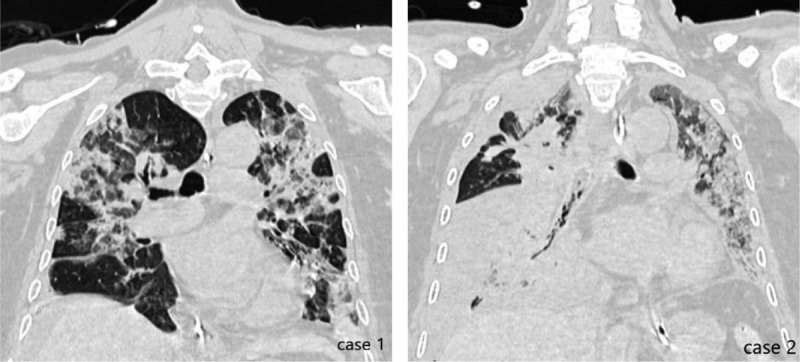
Chest CT scans of case 1 and case 2 when they were intubated for the second time, CT = computed tomography.
